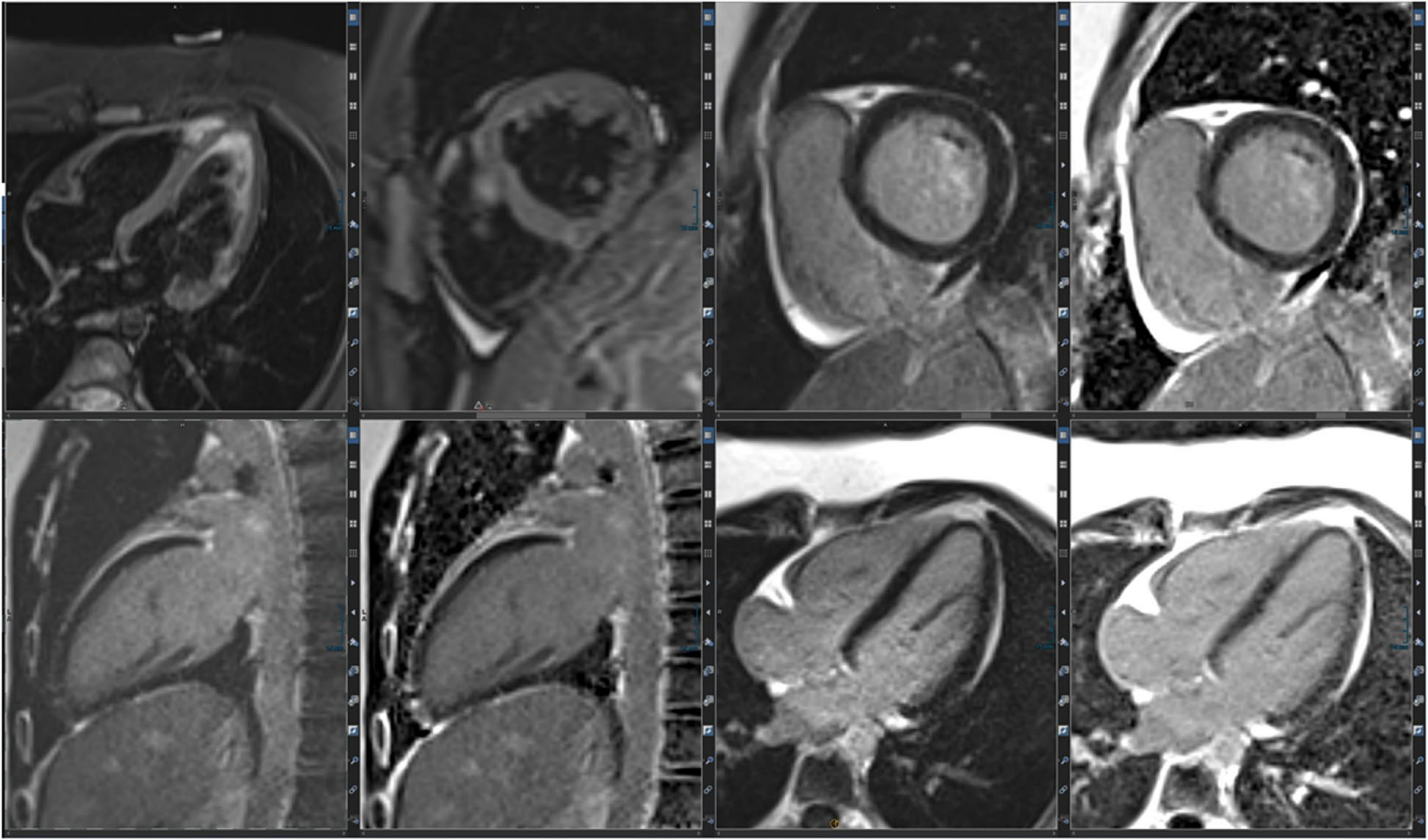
(Tissue integrity assessment by T2 and LGE imaging): the capability of a non-invasive complete assessment of tissue integrity (edema yes/no, fibrosis yes/no) is one of the hallmarks of MRI. Healthy myocardium shows a dark (“hypointense”) signal in T2 imaging (first two images in the left upper row) as well as a dark signal in LGE imaging (right upper row LGE-SAX in IR and PS reconstruction; lower row—LV 2CV- and 4 CV-LGE)
